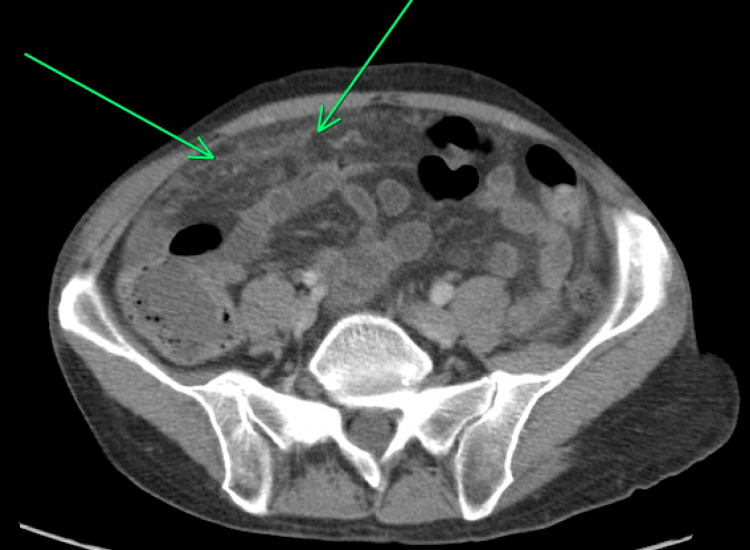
computed tomography (CT) abdomen and pelvis with contrast prominent nodularity of the mesentery (green arrows) which may represent venous congestion from portal hypertension versus peritoneal carcinomatosis.
